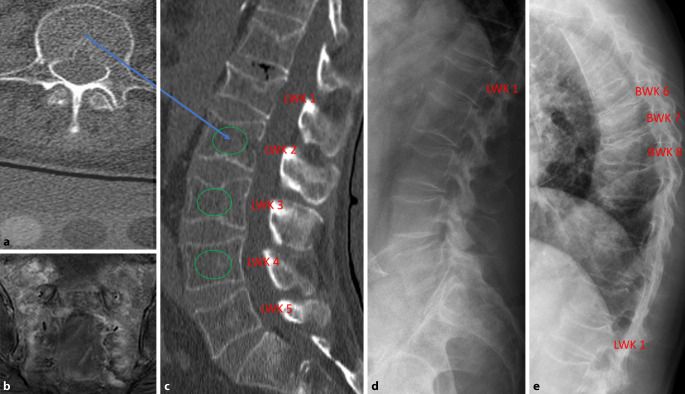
a Mittvertebrales axiales CT-Schnittbild mit darunterliegendem Referenzkörper für die lumbale Osteodensitometrie. b Koronares MRT-Schnittbild (STIR-Sequenz) mit Darstellung einer beidseitigen frischen Sakrumfraktur. c Sagittal reformiertes CT-Schnittbild mit eingezeichneter ellipsoider „region of interest“ in die nicht frakturierten Lendenwirbelkörper LWK 2, LWK 3 und LWK 4 (*grüne Kreise*). d und e laterale Röntgenaufnahme der Lendenwirbelsäule und Brustwirbelsäule zur Detektion der osteoporotischen Wirbelkörperfraktur. Bei der 78-jährigen Pat. ergab die lumbale Bestimmung mittels quantitativer Computertomographie einen Knochenmineralgehalt (KMG) von 30,8 mg/cm^3^ als Mittelwert aus LWK 2, LWK 3 und LWK 4, dieses ist Ausdruck einer schweren Demineralisation. Die spongiöse Dichtemessung ergab einen Mittelwert von 27,8 HU und nach Umrechnung ein KMG von 31 mg/cm^3^, welches sehr gut mit der gemessenen Knochendichte korrespondiert; *BWK* Brustwirbelkörper
